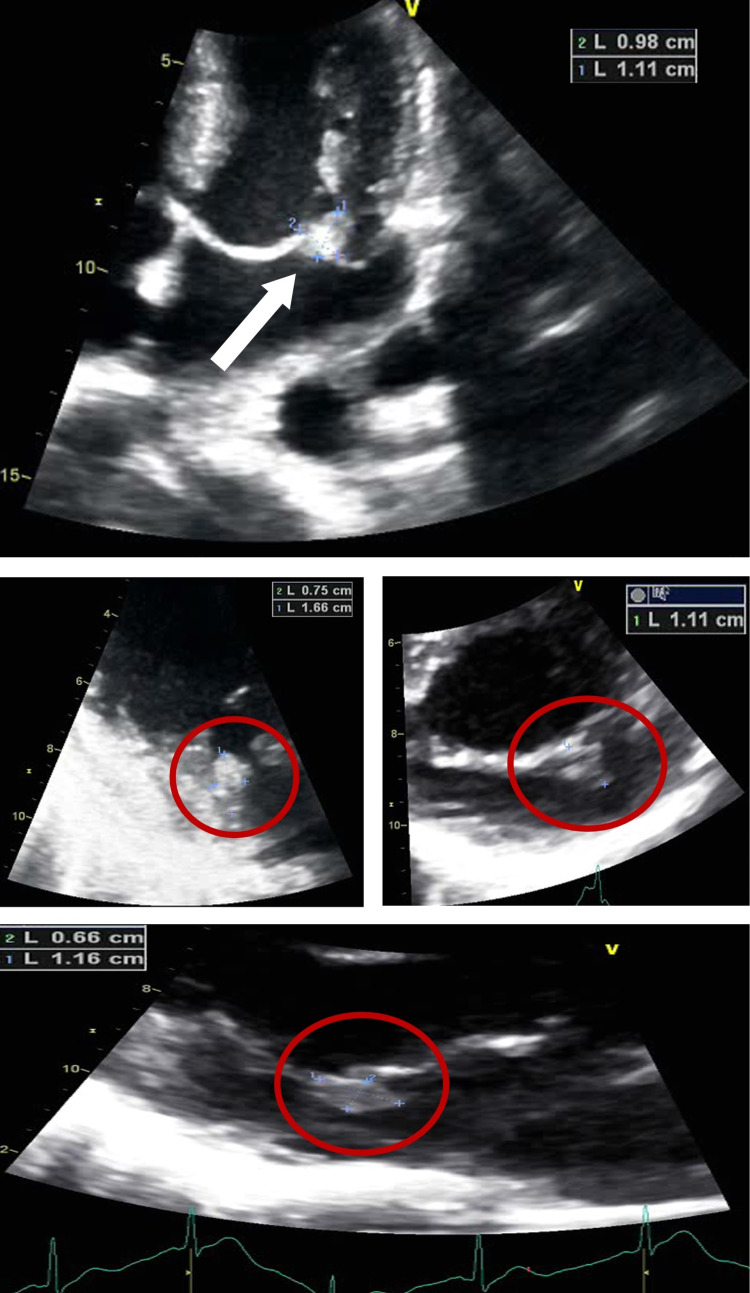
Echocardiographic imaging showing anterior leaflet mitral valve vegetation roughly measuring 1.66 cm x 0.98 cm (white arrow and red circles).
